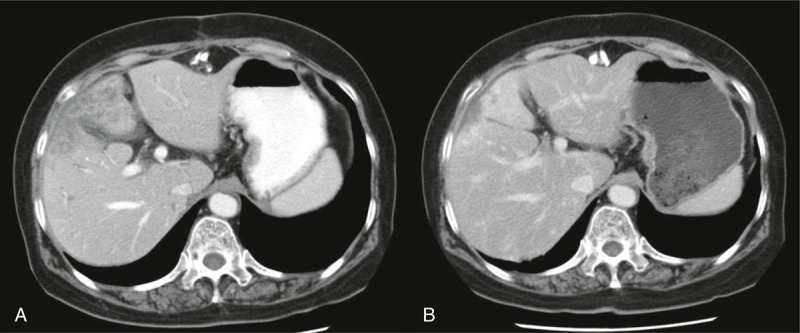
Abdominal CT scan before and after gemcitabine/doxifluridine combination chemotherapy. (A) Baseline CT finding. (B) Three weeks after 4 cycles of the combination chemotherapy.
